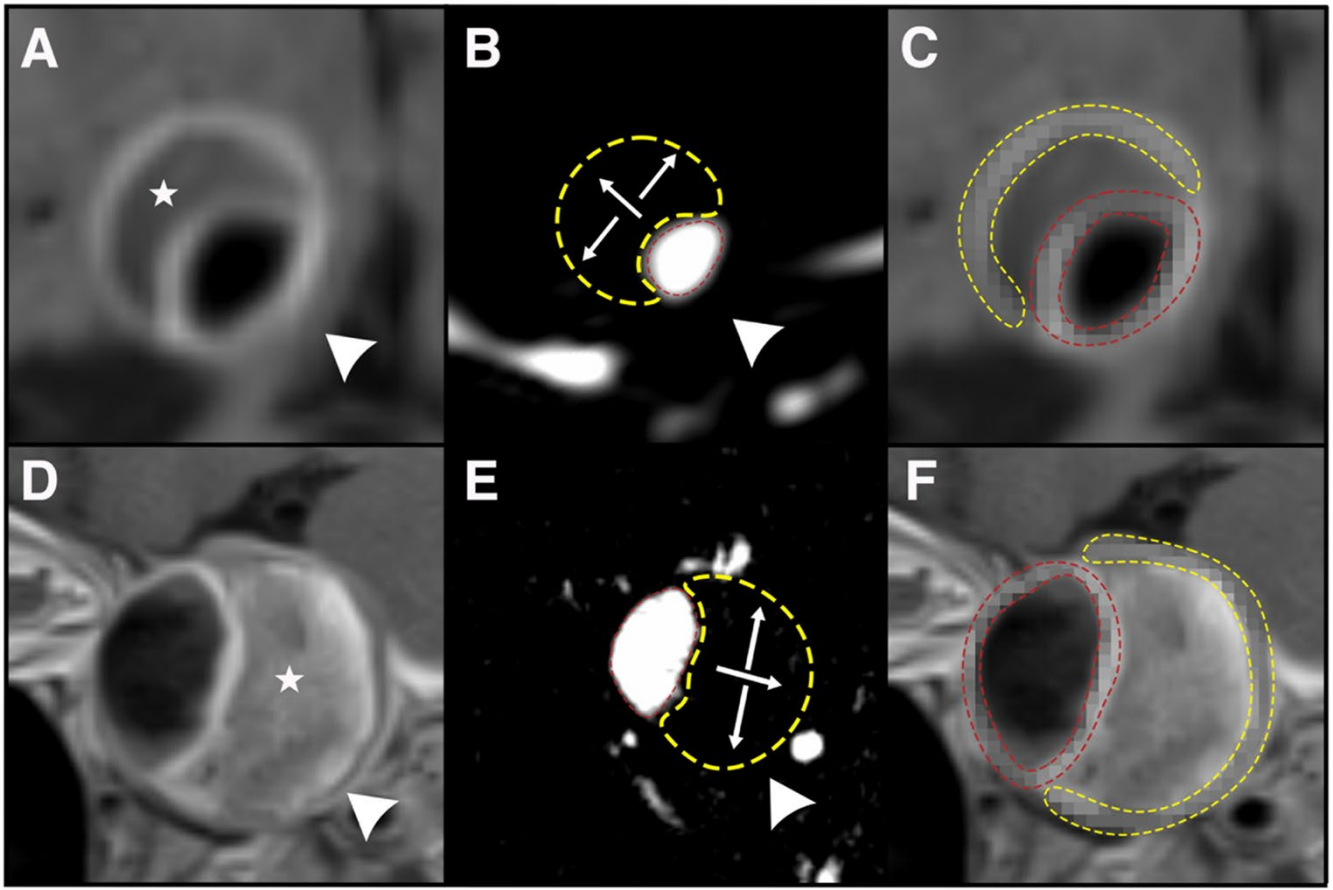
A terminal internal carotid artery (ICA) saccular aneurysm (A) with intrasaccular thrombosis (white star) is identified (arrowhead) in high-resolution magnetic resonance imaging (HR-MRI). Likewise, a (D) cavernous ICA fusiform aneurysm with IST (white star) is visualized in HR-MRI (arrowhead). (B & E) The boundaries of the thrombus (white arrows, yellow circle) are then identified on magnetic resonance imaging (MRA). (C, F) The wall next to the thrombus (yellow circle) and free of thrombus (red circle) is isolated, and radiomics are extracted
